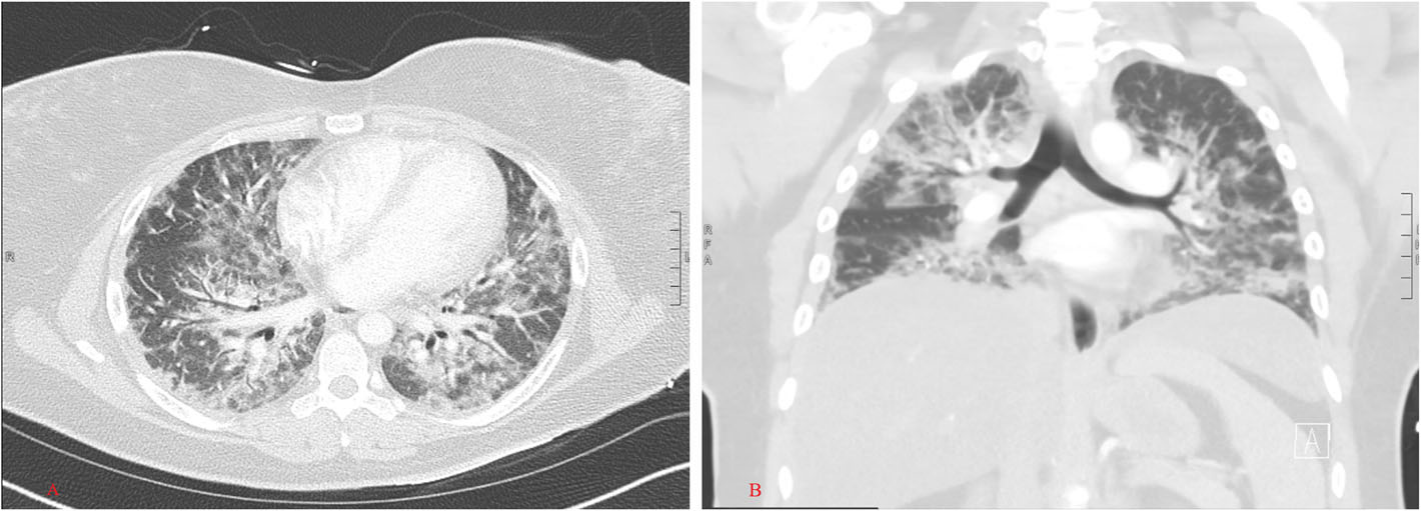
a-b: CT of the thorax showing diffuse ground glass opacities at the axial view (1a) and at the coronal view (1b)
